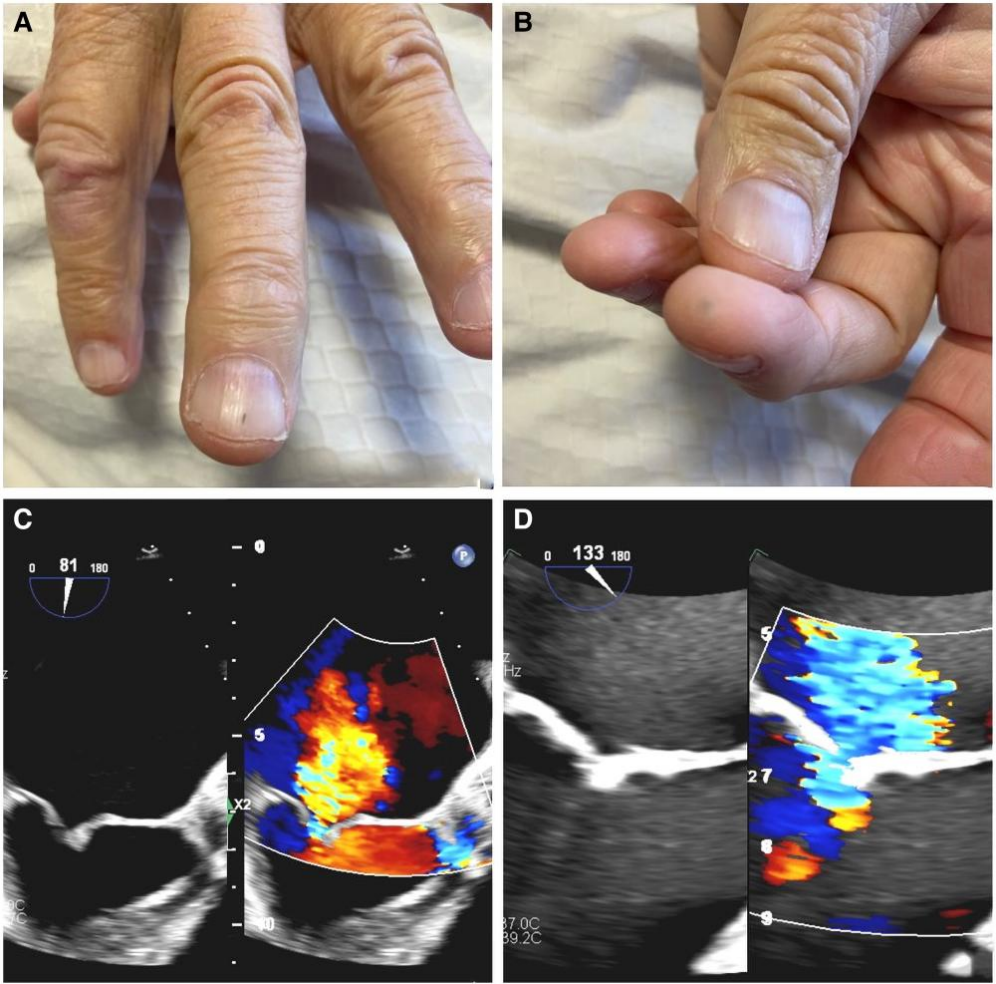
Clinical and echocardiographic findings in a patient with infective endocarditis. (*A*) Splinter haemorrhage and (*B*) Janeway lesion observed during the initial clinical presentation. (*C*) Initial transoesophageal echocardiography demonstrating thickened mitral valve leaflet tips (5.5 mm) with known moderate mitral regurgitation. (*D*) Follow-up transoesophageal echocardiogram showing no significant change in leaflet thickness or degree of mitral regurgitation. Nyquist limit was adjusted for MR quantification; image selection was based on availability and optimal visualisation of the MR jet.
